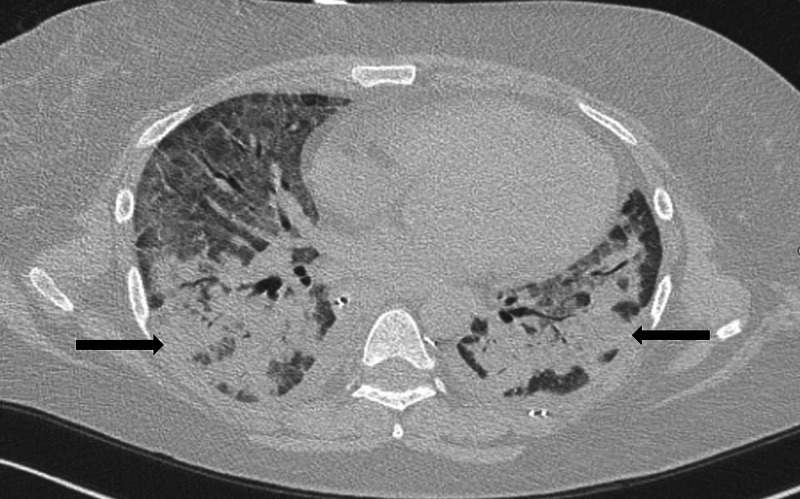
Bilateral ground-glass opacities seen on chest CT (black arrows) CT: computed tomography
